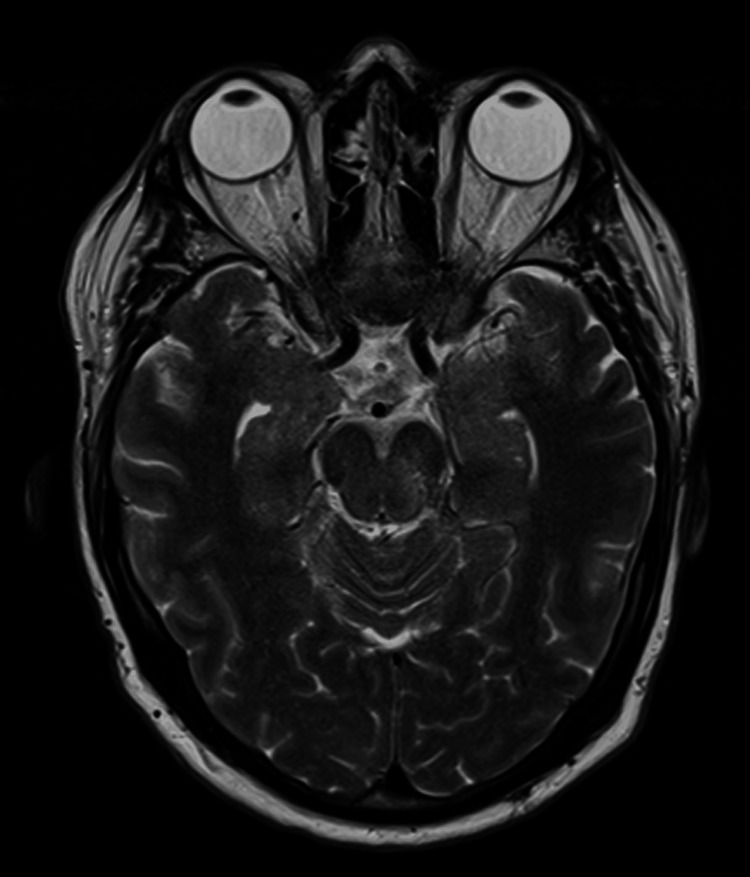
MRI brain showing areas of abnormal enhancement within the basilar cisterns (T2 FLAIR sequence, transverse plane). FLAIR: Fluid attenuated inversion recovery.
